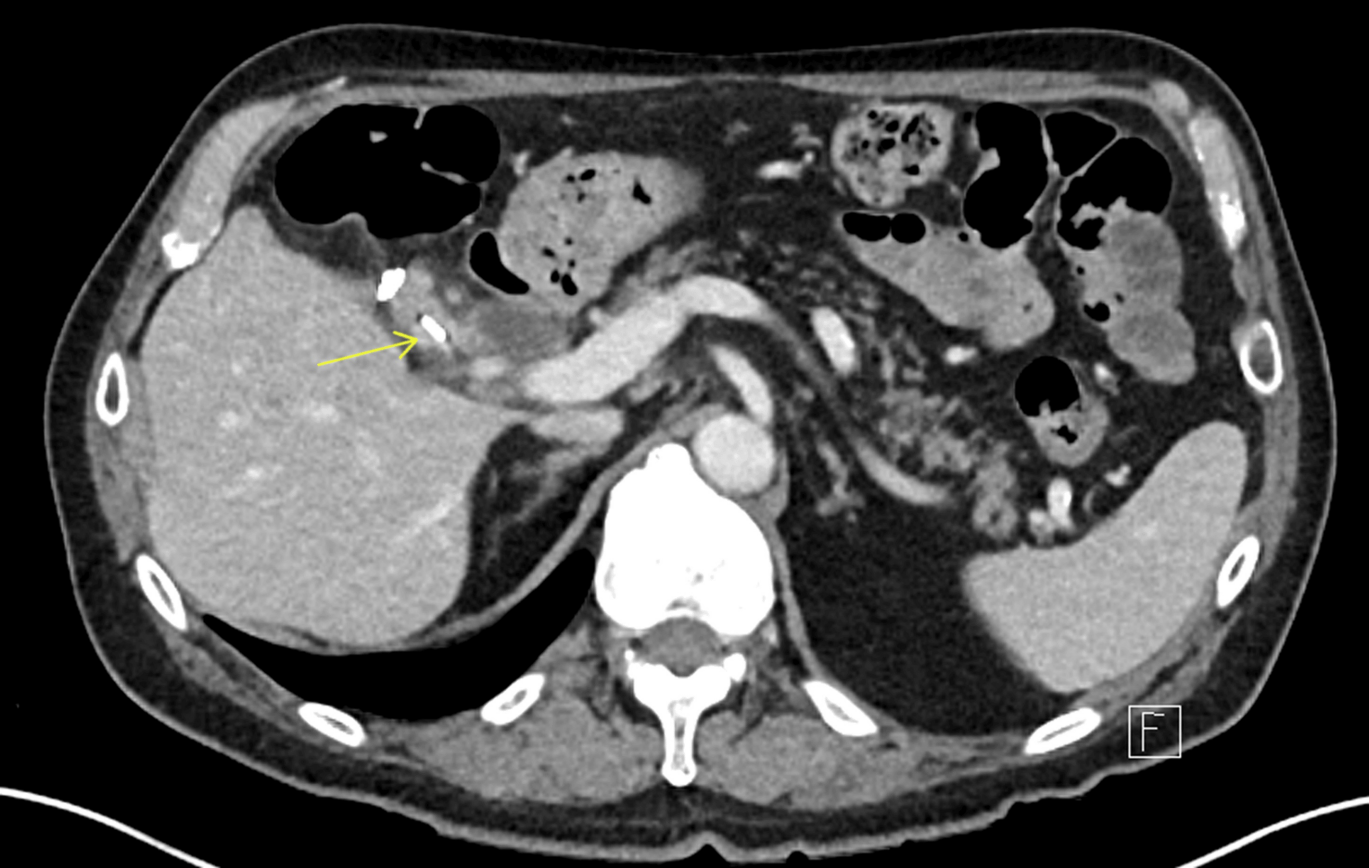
Axial CT from the patient's second presentation to the hospital showing migration of the surgical clip to the remnant cystic duct. Inflammatory stranding can be seen surrounding the migrated clip.
